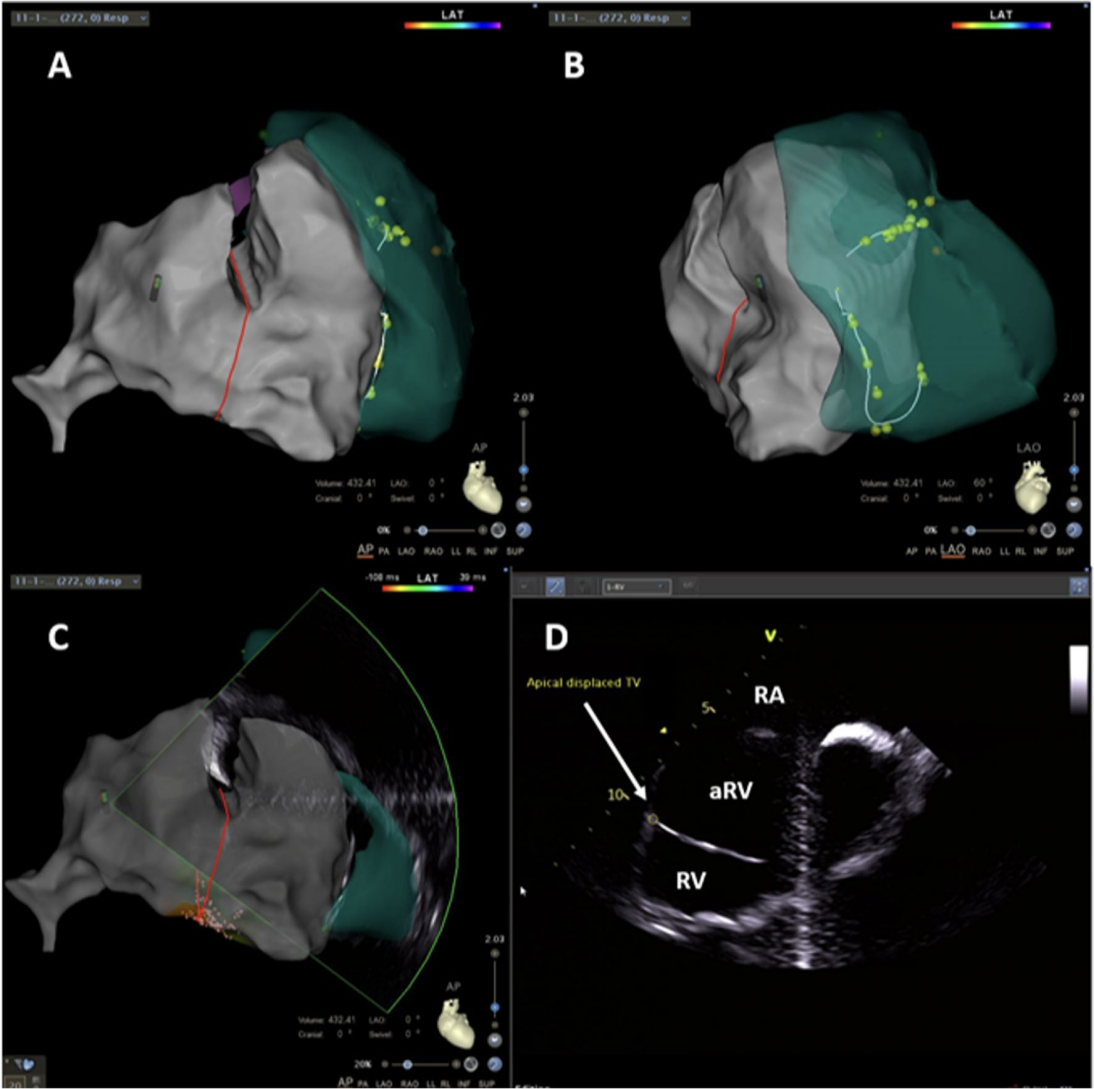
Images using CARTO system (Biosense Webster) with intracardiac echocardiography. A Vision Wire was placed in the right coronary artery to mark the plane of the true annulus. Panels A and B represent the AP and the LAO views, respectively. Panel C shows the plane of the ultrasound beam used to derive the image in the panel D. The red line denotes the plane of the tricuspid annulus, while the yellow tags and the connecting white line marks the plane of the displaced tricuspid leaflet (arrow in D). Also marked are the right atrium (RA), the atrialised right ventricle (aRV), and the right ventricle (RV)
